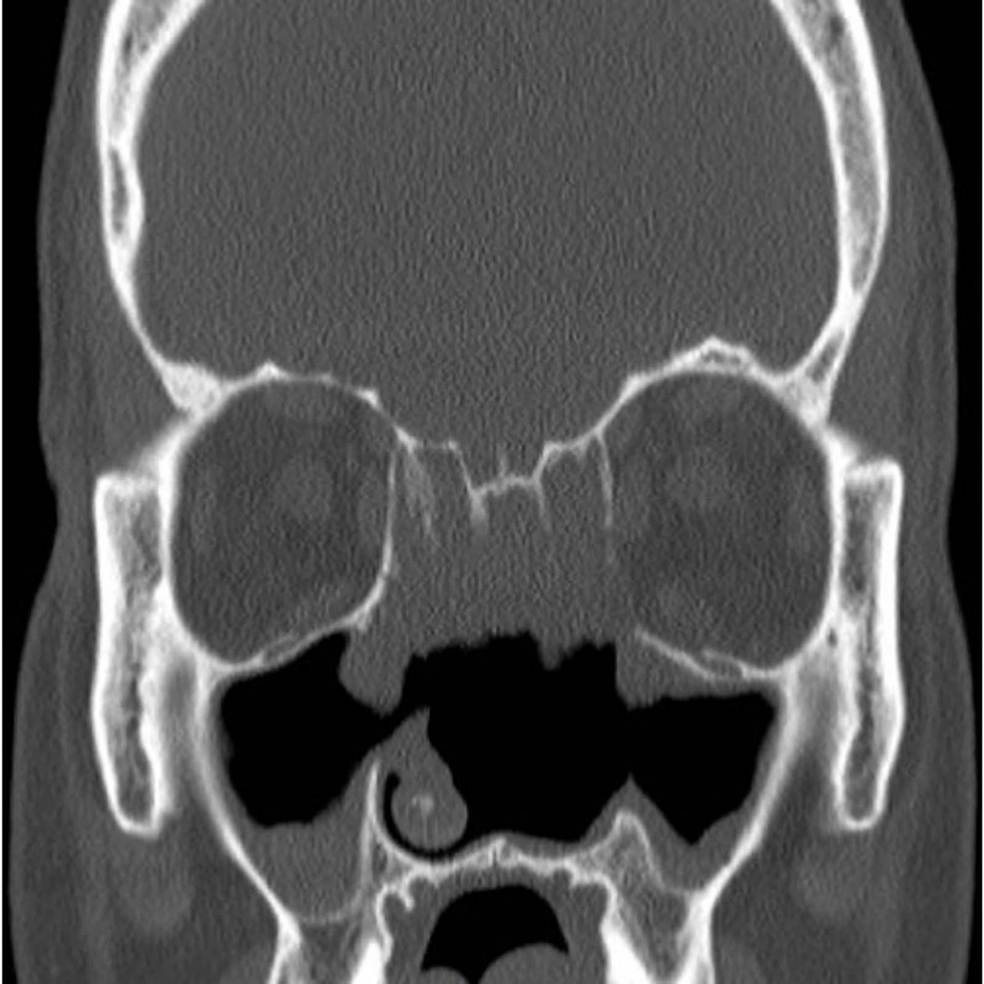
Postoperative CT scan: coronal view shows no residual masses.
